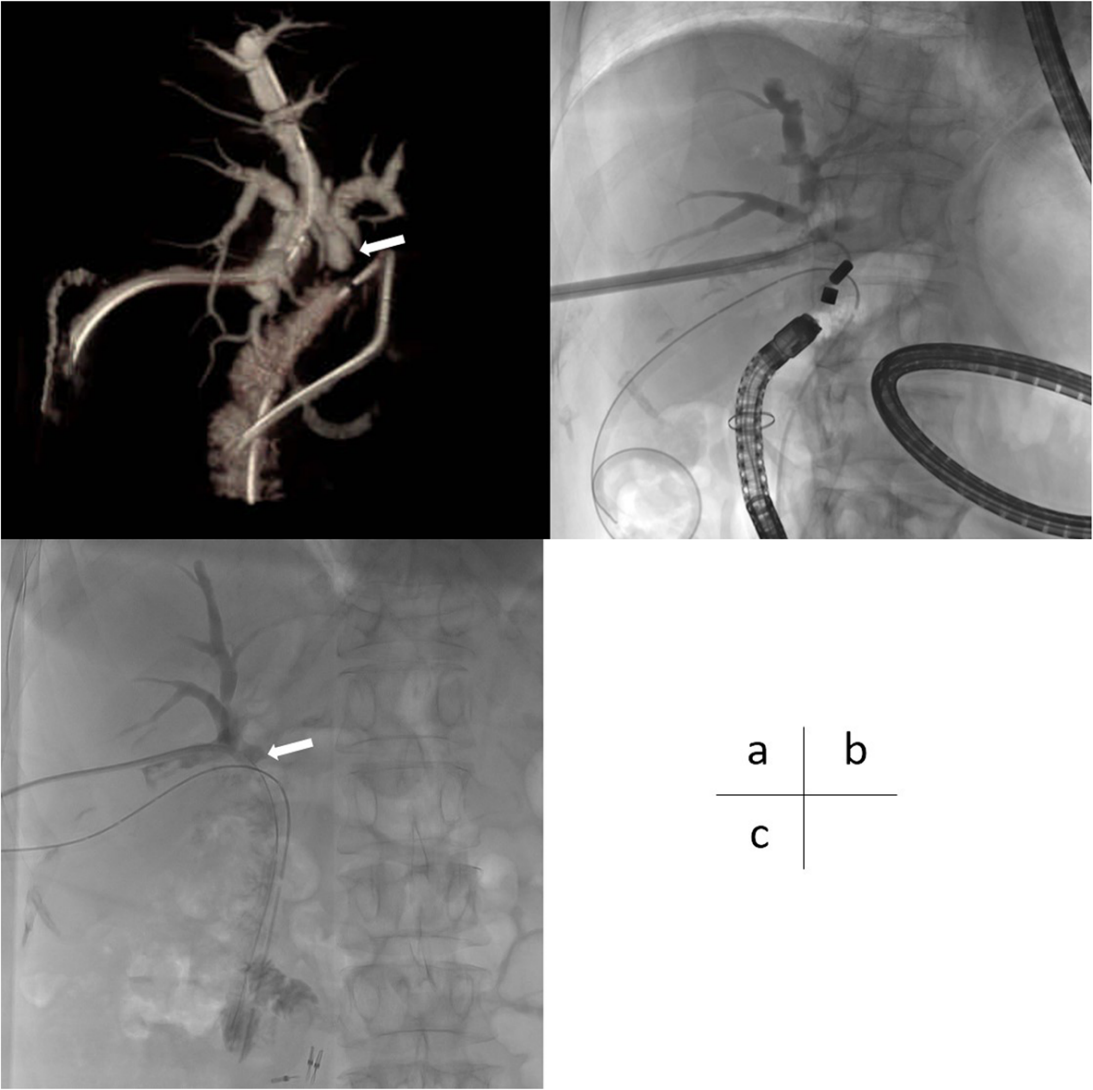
a We checked the stumps of the RPD (white arrow) by 3D reconstruction of CT image. b MCA for the RPD was performed by the same procedure as that for the RAD. c The bilioenteric fistula of the RPD was completed 47 days after MCA. The PTCD tube was introduced into the bowel to maintain the fistula through re-anastomosis of RPD (white arrow). *MCA* magnetic compression anastomosis, *RAD* right anterior duct, *RPD* right posterior duct
